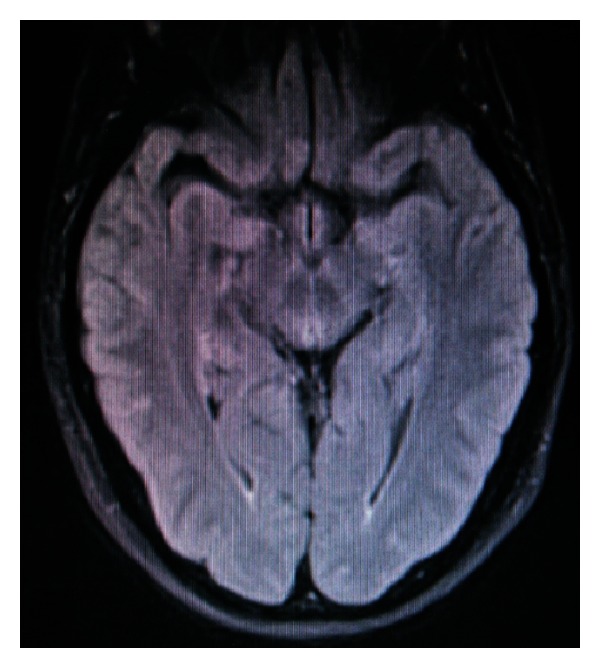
Fluid attenuated inversion recovery magnetic resonance imaging at follow up (15 days after the event) indicating that all lesions have disappeared.
